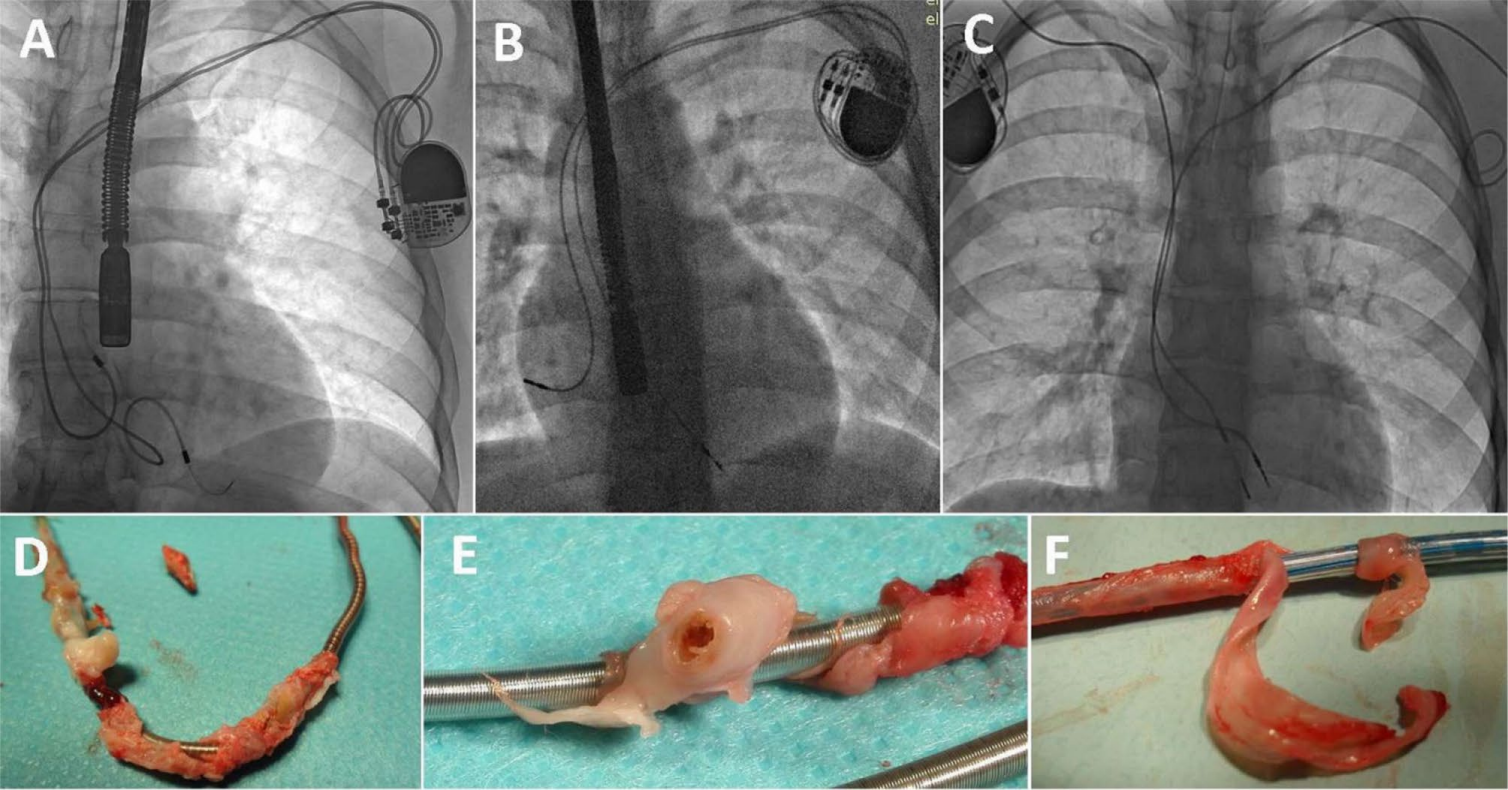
Several examples of X-ray and view of extracted leads. (A) Planned to long lead loops in the heart implanted 20 years before TLE. (B) Strained (“standing”) 12 y old dysfunctional RV lead due to body growth. RA lead was added 5 year ago during system upgrading. (C) 18-y old VVI pacing system (R) and abandoned 15-y old RV lead. (D) Strong advanced connecting tissue scar surrounding distal part of RAA lead. (E) Extracted in young adult ICD lead; strong massive scar (tunnel form) connecting two of leads before extraction—visible on one of two extracted leads. Such phenomenon make extraction more difficult. (F) Another form of (more floppy) scar on extracted ICD lead in young adult.
